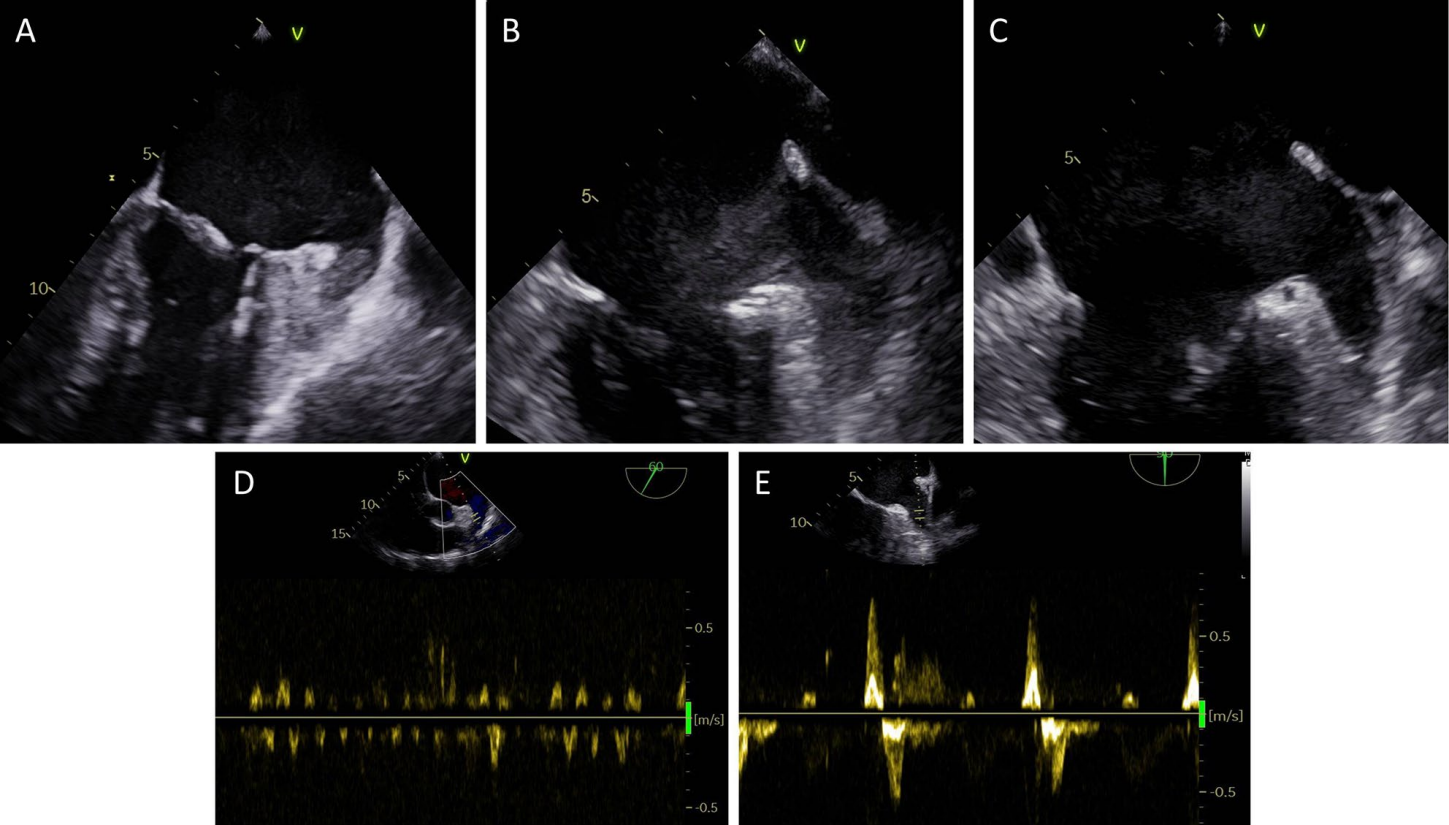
Representative images of a solid LAA thrombus (A), LAA sludge (B), not containing a solid thrombus on i.v. contrast imaging), and a LAA free of thrombus or sludge (C). (D) shows the PW Doppler signal in a patient with LAA emptying velocity reduced ≤ 20 cm/s while panel (E) shows a LAA with normal flow characteristics.
